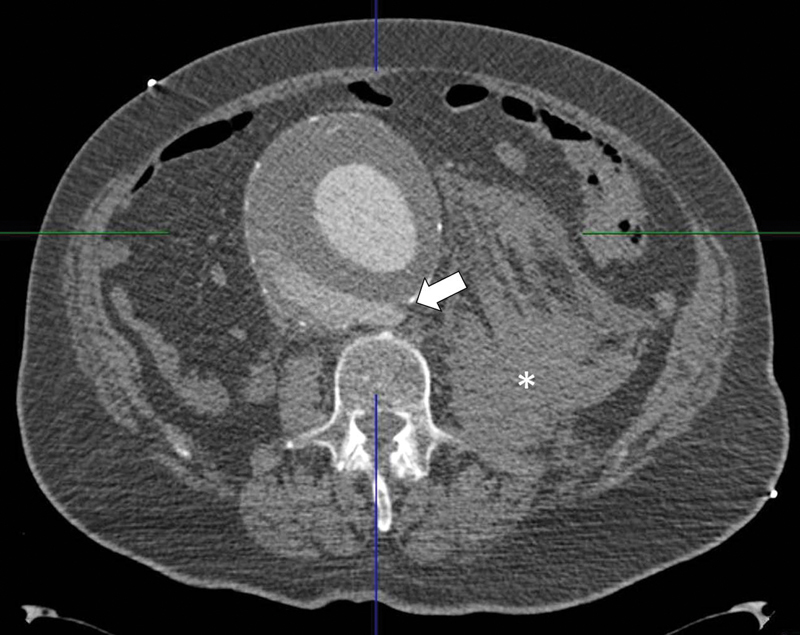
Computed tomography angiogram showing a voluminous abdominal aortic aneurysm (11.5 cm in diameter) with rupture of posterior aortic wall (white arrow) and intra-abdominal hematoma (white asterisk) on the left retroperitoneal region.
